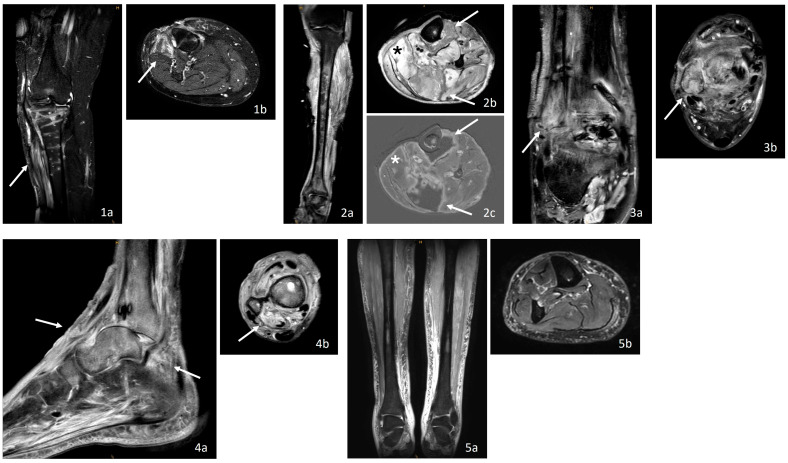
MRI findings in controls. Soft tissue edema with contrast enhancement (↑), also see Table 4. (1) MRI of the right lower leg with soft tissue infection without bone marrow edema ((a): coronal, STIR; (b): transverse, T2w mDIXON TSE). (2) MRI of the left lower leg with soft tissue infection and muscle necrosis (*) ((a): coronal, STIR; (b): transverse, T2w mDIXON TSE; (c): transverse, sT1w TSE). (3) Non-specific MRI of the right ankle with soft tissue infection and contrast enhancement in the distal fibula ((a): coronal, PDw mDIXON TSE MARS; (b): transverse, PDw mDIXON TSE MARS). (4) Non-specific MRI of the right ankle with soft tissue infection and subtle contrast enhancement in the adjacent bone ((a): sagittal, PDw mDIXON TSE; (b): transverse, PDw mDIXON TSE). (5) MRI of the right lower leg with soft tissue infection without bone marrow edema ((a): coronal, STIR TSE; (b): transverse, T2w mDIXON TSE). MARS: metal artifact reduction sequence, mDIXON: modified DIXON, PDw: proton density-weighted, STIR: Short Tau Inversion Recovery, TSE: turbo spin echo, T1w: T1-weighted, T2w: T2-weighted.
